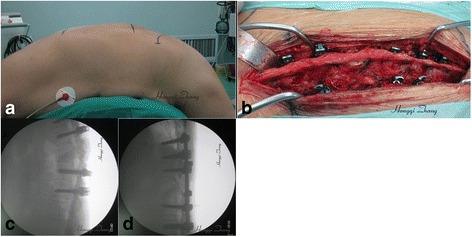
Surgical procedure of osteotomy through the fracture gap in a female patient with ankylosing spondylitis. a Photograph shows the position of the patient on the operating table. The patient was flexed in a reverse V shape to accommodate the kyphotic spine and adapt simultaneously to the correction of kyphotic deformity during operation. b Photograph shows a hyperplastic osteophyte located at the oseteotomy site. c, d Intraoperative X-ray fluoroscope was used after inserting the pedicle screws and correcting the kyphotic deformity
